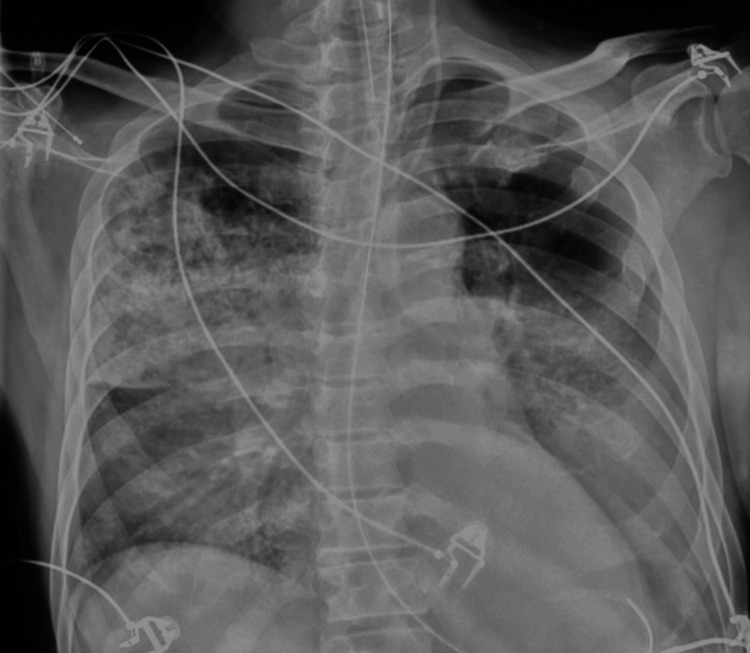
Chest X-ray on the day of admission indicates bilateral, predominantly right-sided strip-like patchy pneumonia infiltrates, as well as decreased transparency in the left basal region, where a small amount of pleural effusion was verified by ultrasound.
